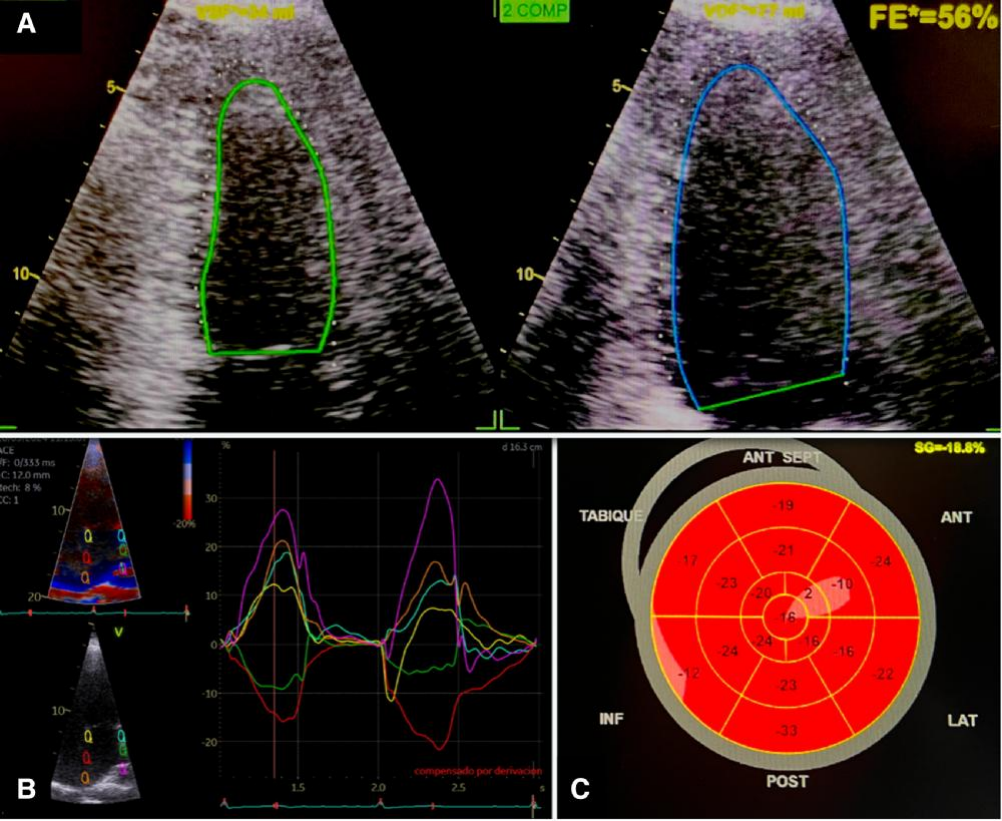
Transthoracic echocardiogram. (*A*) Preserved left ventricular ejection fraction. (*B*) Left atrial strain of 16%. (*C*) Left ventricular strain of −18.8%.
